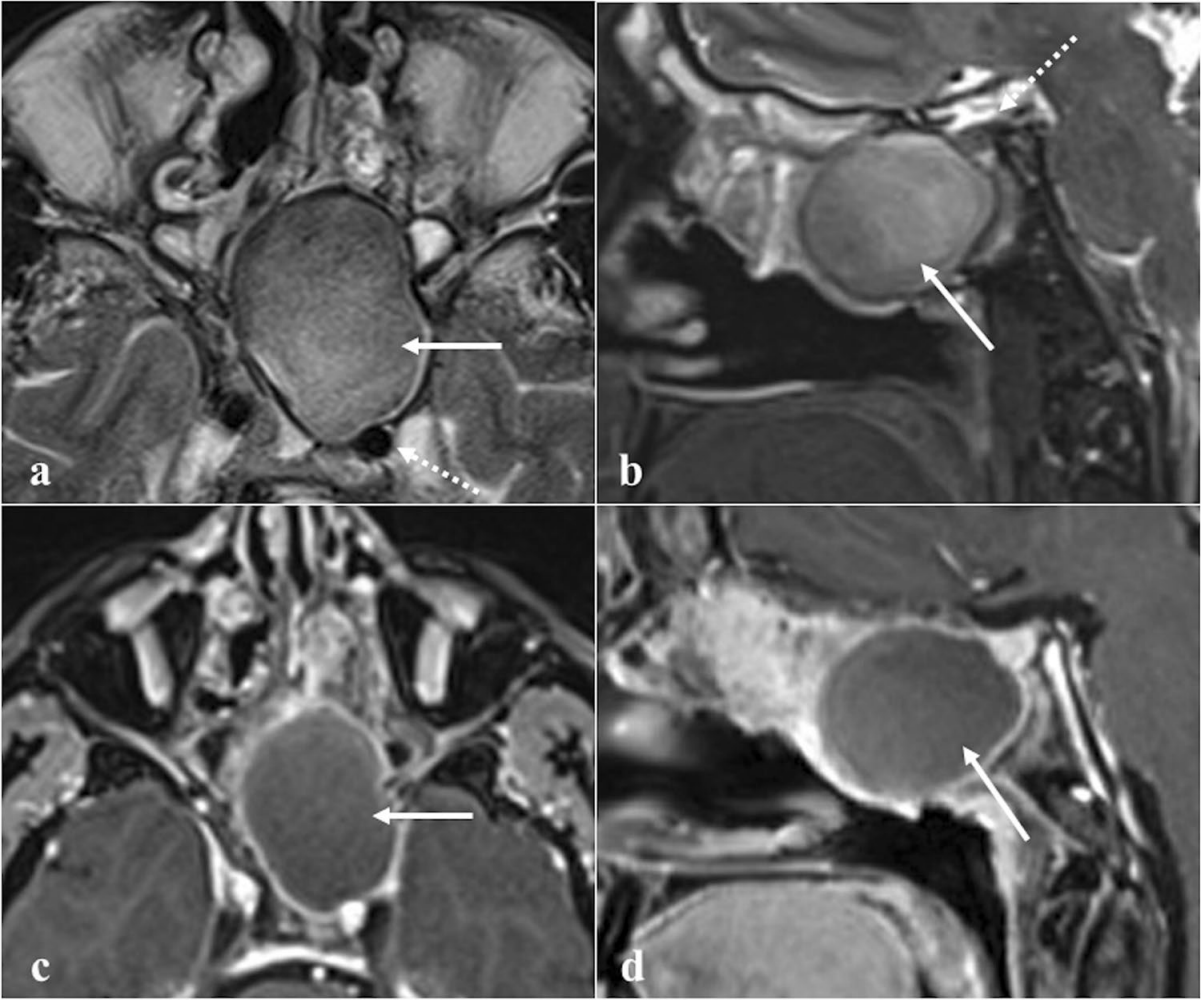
Left sphenoid sinus mucocele with high protein content in a 53-year-old male patient with headache. MRI shows a large mass (white arrows) displacing the ipsilateral internal carotid artery posteriorly (white dotted arrow) on T2W axial image (a) and the pituitary gland superiorly (white dotted arrow) on T2W sagittal image (b). Sphenoid sinus is markedly enlarged with mucous content and peripheral rim enhancement on axial (c) and sagittal (d) T1W fat-saturated CE images. No sign of superimposed infection or invasion of the adjacent structures is observed
